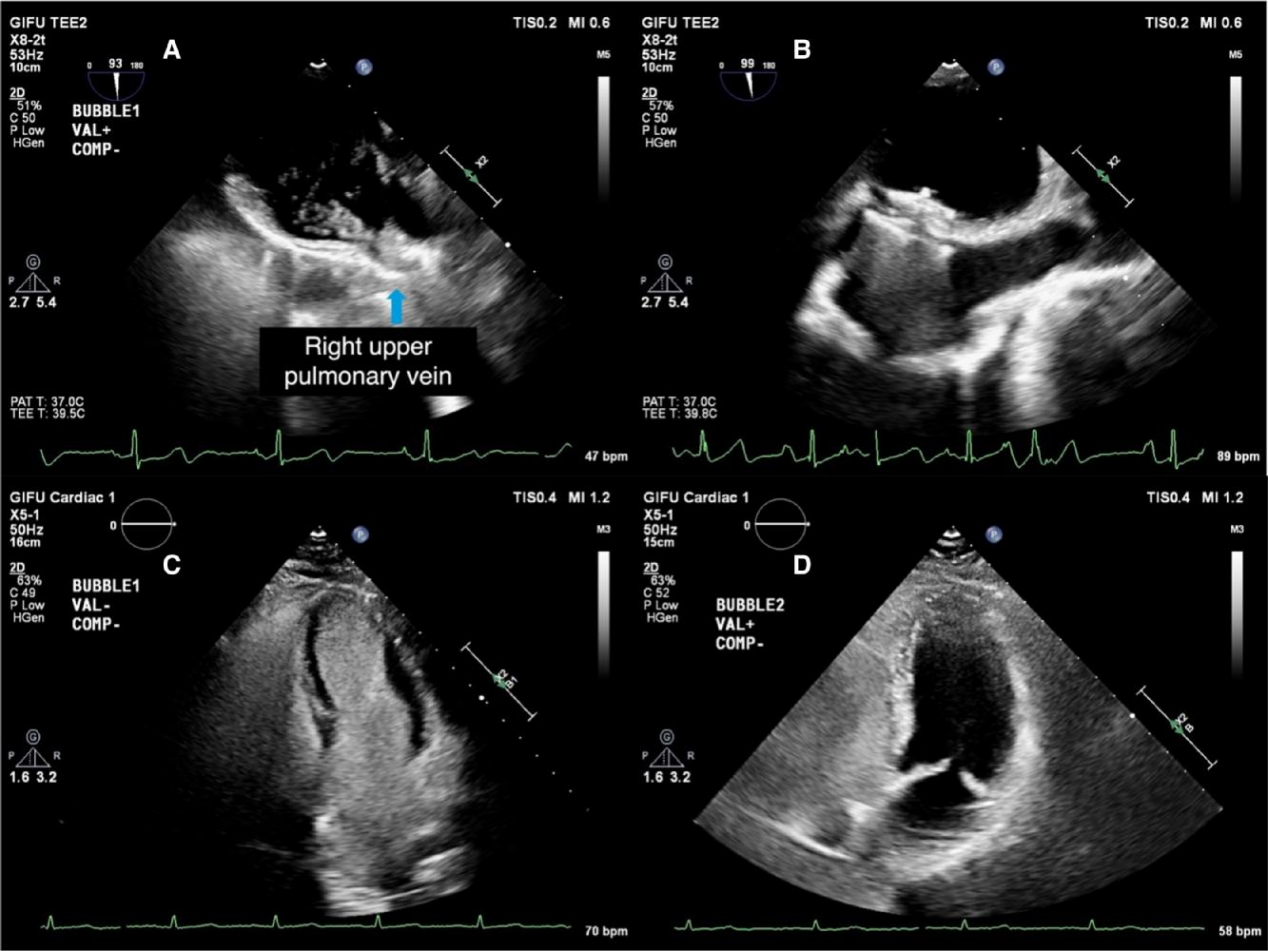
Intraoperative transoesophageal echocardiography and follow-up transthoracic echocardiography during patent foramen ovale and pulmonary arteriovenous malformation closure. (*A*) Intraoperative transoesophageal echocardiography: grade 3 bubble flow from the right upper pulmonary vein to the left atrium. (*B*) Intraoperative transoesophageal echocardiography: post-patent foramen ovale closure with occlusion device. (*C*) Transthoracic echocardiography post-patent foramen ovale closure, pre-pulmonary arteriovenous malformation closure: grade 4 left heart opacification >4 cycles after right heart. (*D*) Transthoracic echocardiography 4 months post-pulmonary arteriovenous malformation closure: no left heart bubble flow.
